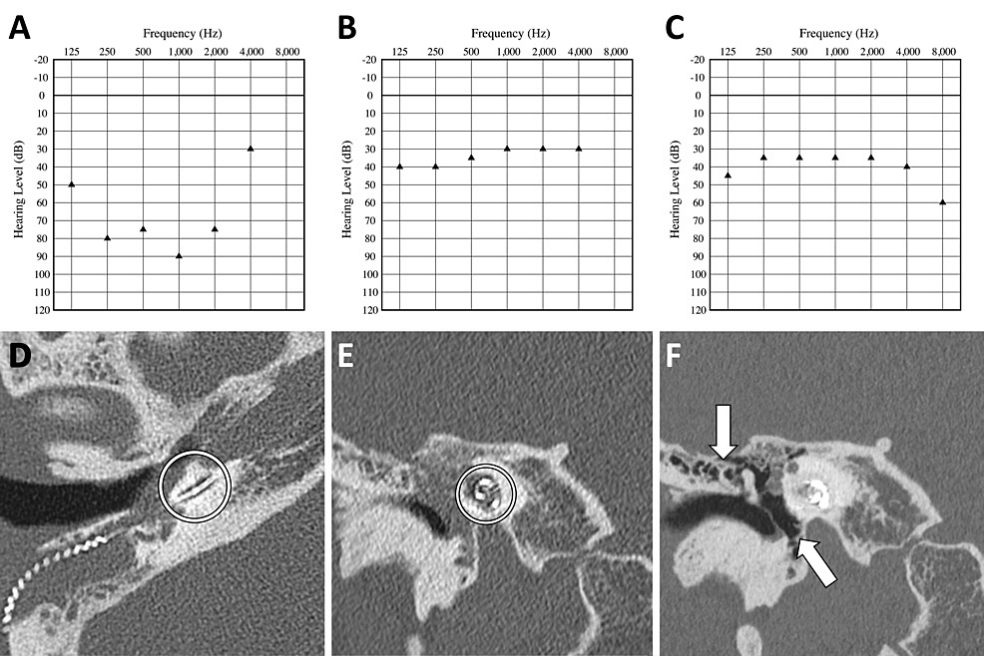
Postoperative clinical findings. (A) Hearing threshold result at five months after cochlear implantation (CI). (B) Hearing threshold result at seven months after CI (one month after introduction of dupilumab). (C) Hearing threshold results at 32 months after CI (25 months after introduction of dupilumab). (D and E) Axial and coronal CT images showing the electrodes correctly inserted into the right cochlea (white circle) and soft tissue density in the right middle ear. (F) Coronal CT image after introduction of dupilumab, showing that the soft tissue density in the right middle ear had disappeared (white arrow).
